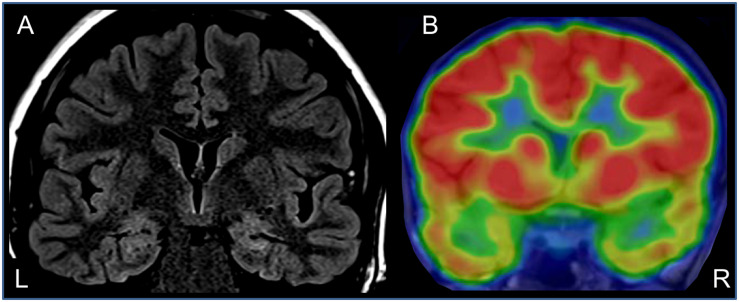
Anatomical and functional neuroimaging – coronal sections through the temporal lobes. (A) T2-weighted FLAIR MRI showing right hippocampal hyperintensity. (B) Interictal brain PET-CT – showing bilateral hypo-metabolism in temporal lobes (right >> left).
